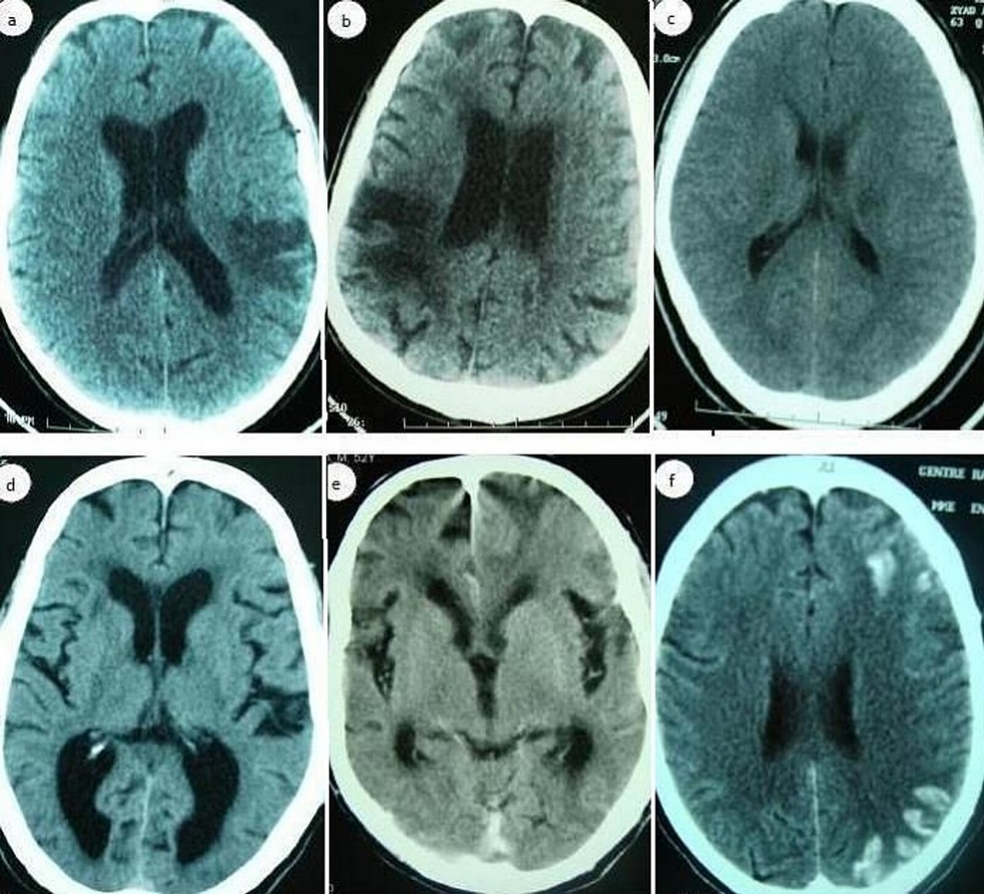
Axial view CT scans; a (patient 2), b (patient 3), note a moderate-to-large well-defined lesion involving white matter and adjacent cortex, conforming to a vascular distribution; c (patient 8), note a focal ischemic lesions in deep area. D (patient 11), e (patient 7), Note a diffuse cerebral atrophy; f (patient 13), note the Meningeal enhancement.
